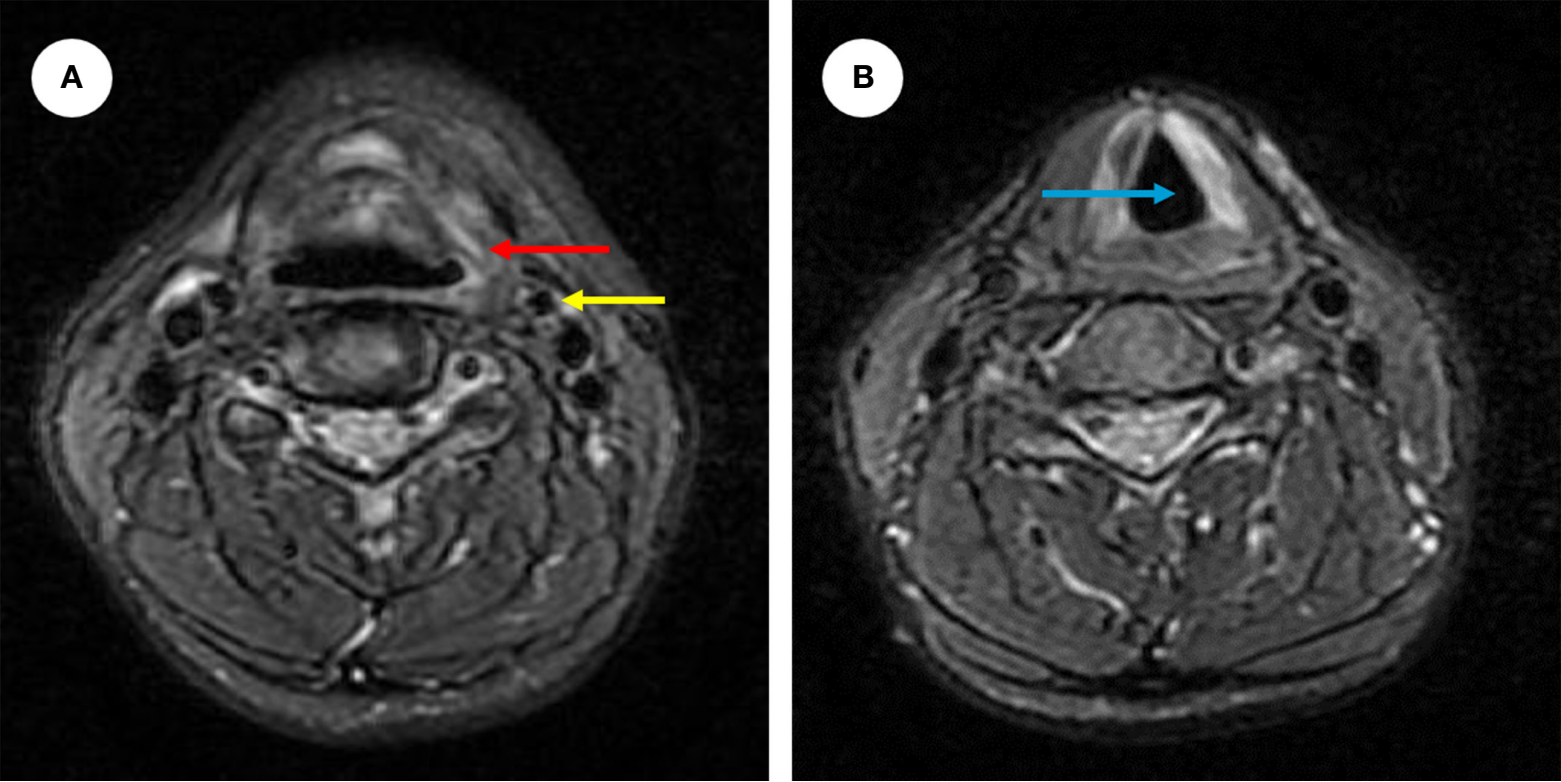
Enhanced MR images showing no tumor recurrence in the precarotid (yellow arrow) and preepiglottic spaces (red arrow) (A) and the normal anatomy of the laryngeal cavity (blue arrow) (B).
